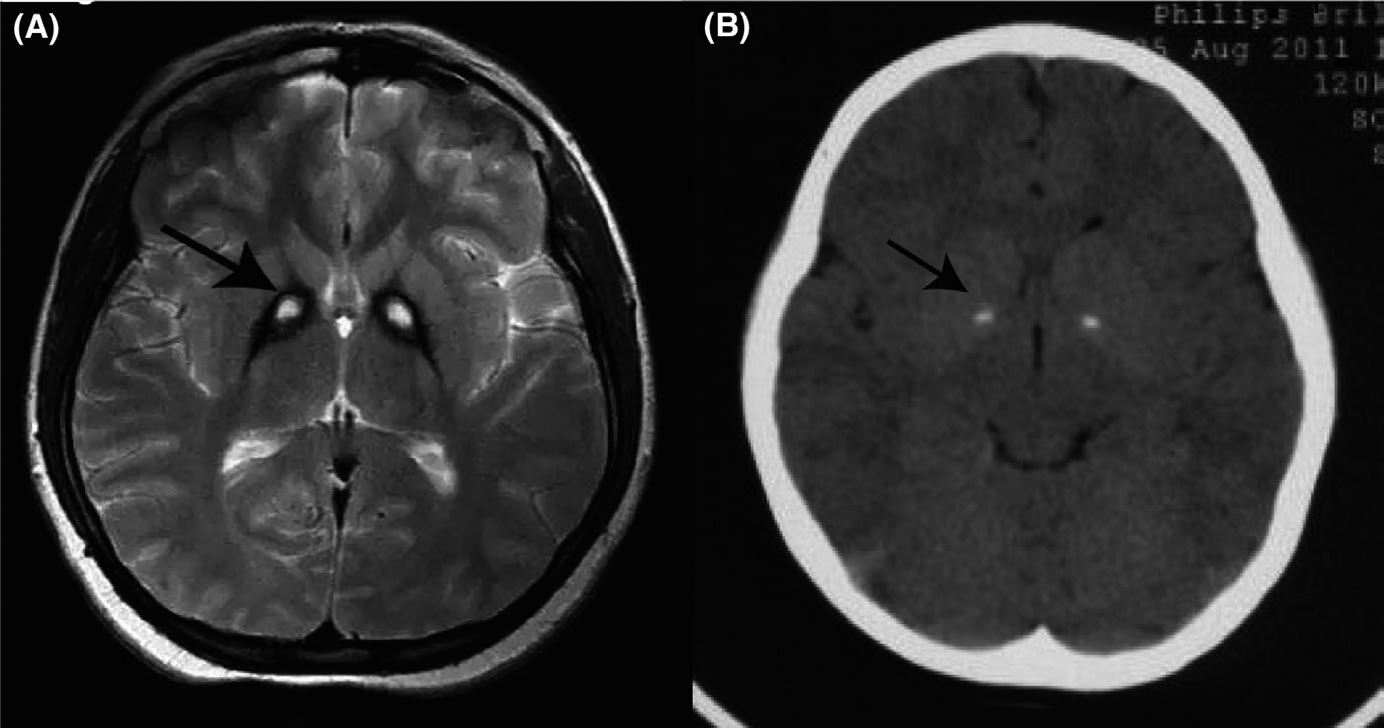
Neuroimaging of patients with PKAN. A, T2‐weighted MRI of the brain showed a specific pattern of hyperintensity (indicated by the arrow) within the hypointense medial globus pallidus (“eye‐of‐the‐tiger” sign). B, Brain CT showed calcification in medial globus pallidus, which was indicated by the arrow
